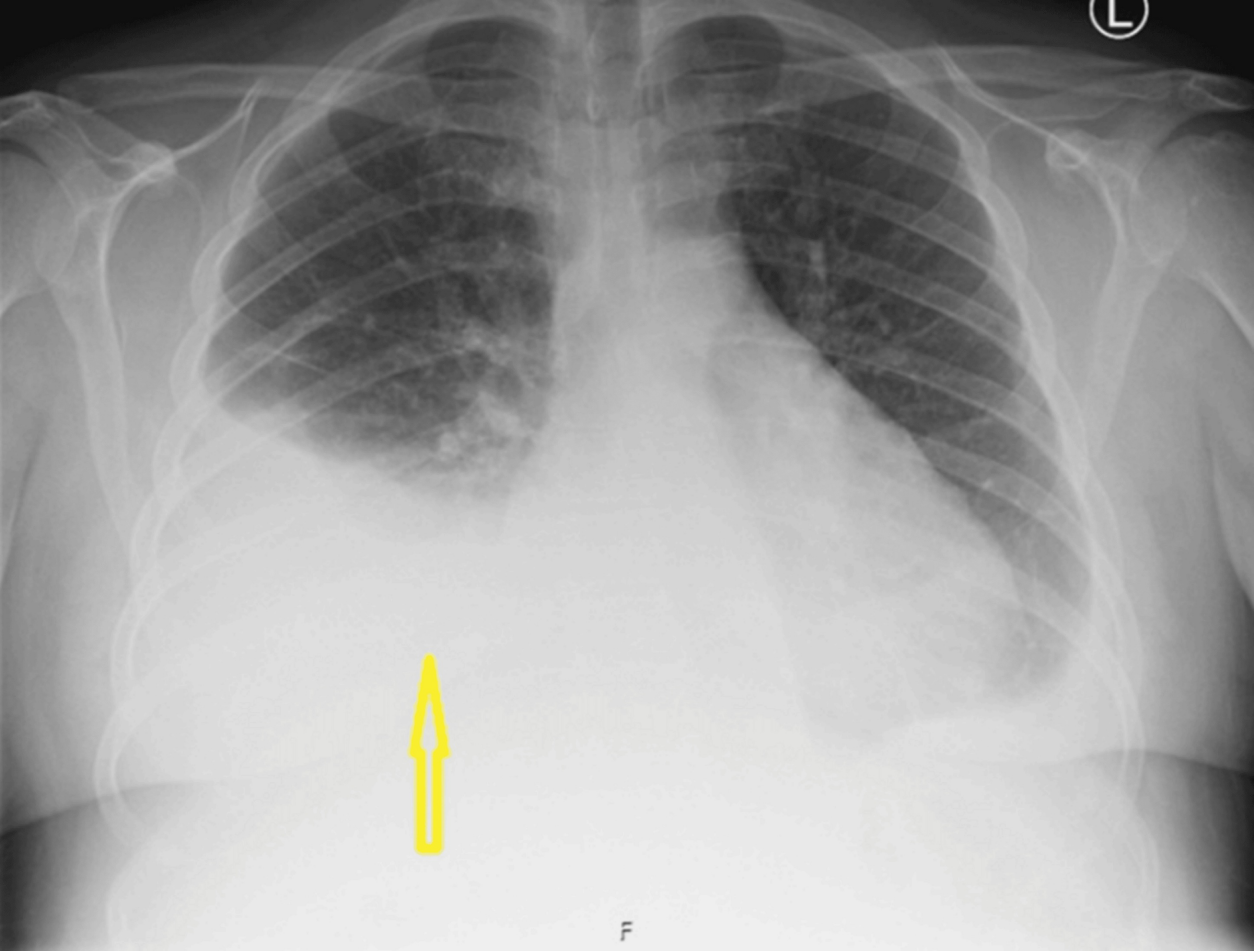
Repeat chest X-ray taken a few months later showing persistent pleural effusions (yellow arrow)
